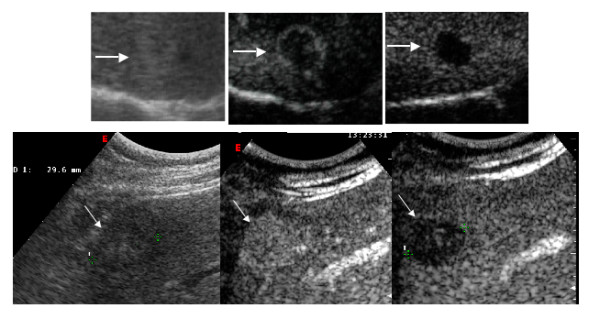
Patterns of liver metastases at CEUS. Lesions are indicted by white arrows. Upper row. In conventional B-mode ultrasound (left frame) the focal liver lesions is hardly visibile, showing a faint hyperechoic apperance. During the arterial phase (23 seconds after contrast injection, central frame) the lesion shows a rim- like hyperechoic aspect, whereas it becomes practically anechoic during the late phase (160 seconds after injections, right frame), consistently with a metastasis from left colonic adenocarcinoma. Lower row: in conventional B-mode ultrasound (left frame) this metastasis from rectal adenocarcinoma appears inhomogeneous, predominantly hypoechoic, whereas it becomes homogeneously hyperechoic during the arterial phase (32 seconds after contrast injection, central frame) and washes contrast out in the late phase, becoming markedly hypoechoic (125 seconds after injection, right frame).
